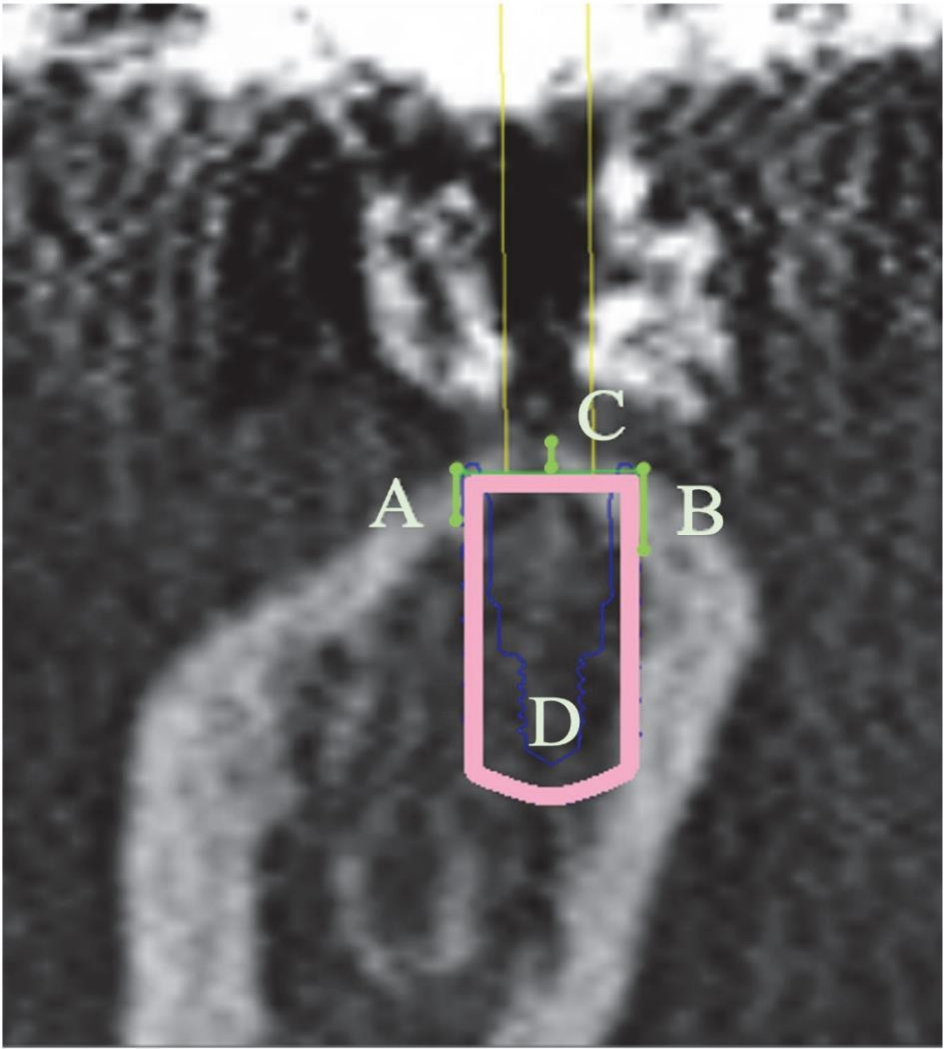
Thickness of the cortical bone at the site of implant placement on each cross-sectional computed tomography image. The cortical bone surface surrounding the implant model was measured at 3 points: the buccal (A), center (C), lingual, or palatal (B) sides, and the trabecular bone CT value of the implant placement site was measured (D).
